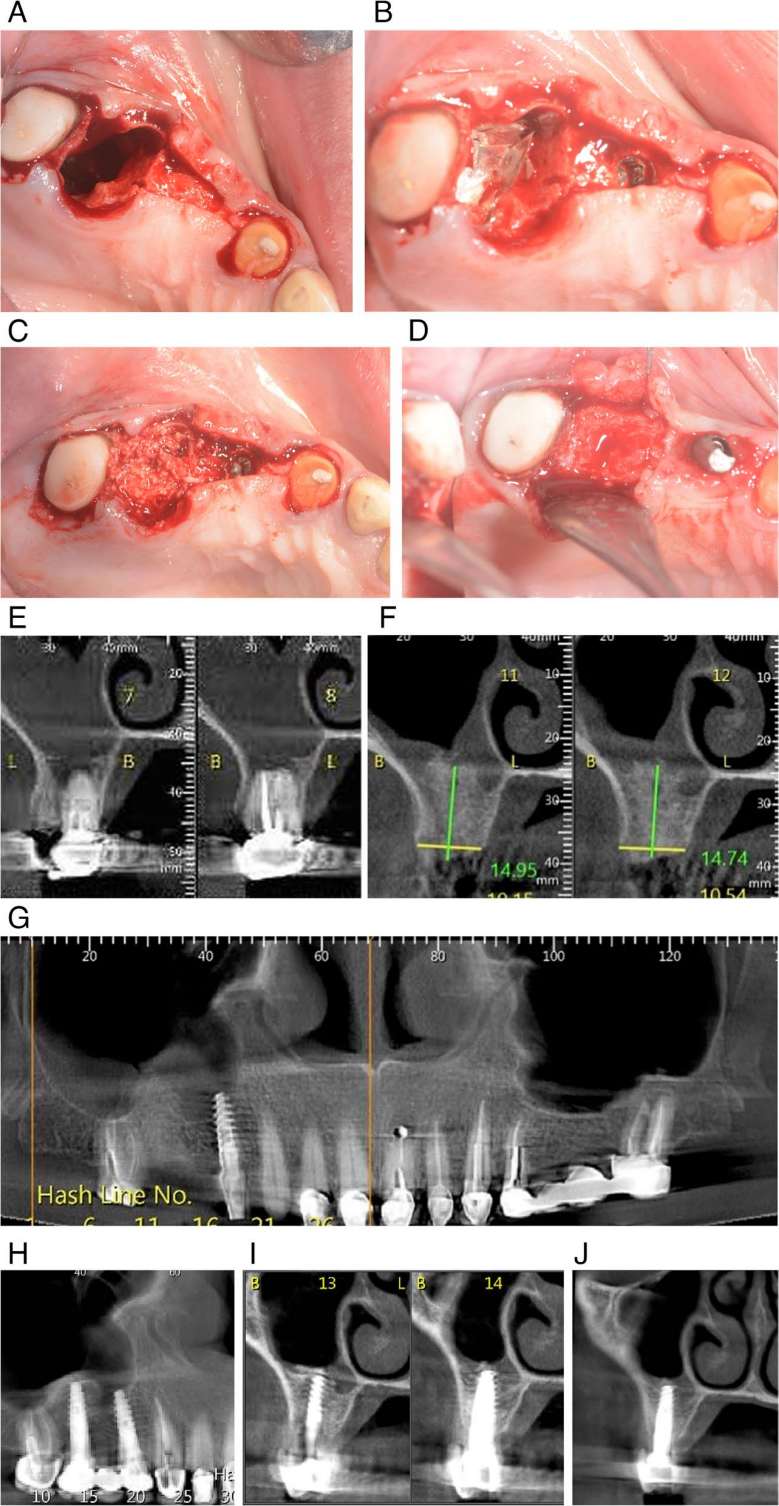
A Tooth 16 was extracted, leaving communication between the oral cavity and the maxillary sinus. B The sulcus was opened through a crestal incision and the dental implant was placed at position 14 (blue arrow). Resorbable magnesium membrane was shaped to close the communication at position 16 (black arrow). C The cavity at position 16 was filled with allograft and xenograft granules, the soft tissue was sutured, and a healing cap was placed on the implant (blue arrow). D Five months post-operatively, the implant was stable, with no abnormal movement, and the graft material was integrated with surrounding healthy hard tissue. E Coronal CBCT section taken before extraction shows that there is no alveolar bone between the apical third of tooth 16, which ends in the right maxillary sinus. F CBCT cross-section taken five months after graft placement shows 15 mm of newly formed bone, sufficient for placement of a new fixed restoration. G Panoramic image taken five months after implant placement at position 14. Newly formed bone tissue has formed adjacent to the implant with no signs of peri-implantitis. H The panoramic image was taken four months after implant placement at position 16 in the maxilla. A dental bridge is attached to implants 14 and 16 as well as healthy tissue around the previously placed implants. I, J Coronal CBCT images slices show correct placement of the implant between the buccal and palatal alveolar walls and the presence of new alveolar bone in the apical third of the implant, creating complete separation from the right maxillary sinus
